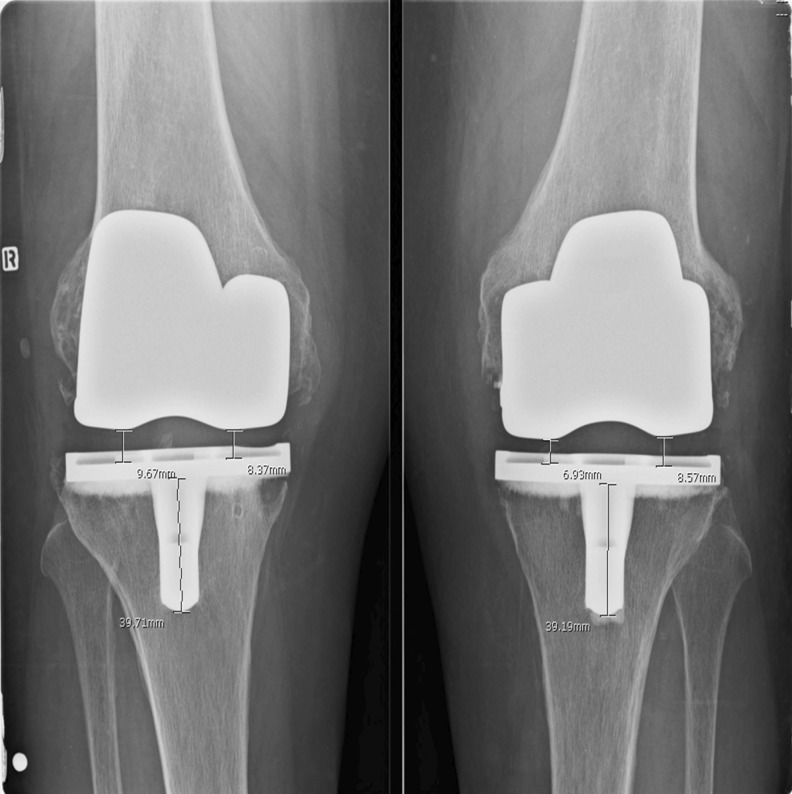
Polyethylene (PE) thickness of medial and lateral compartments measured on anteroposterior radiographs. The estimated amount of linear wear was calculated by deducting the measured thickness, corrected for magnification, from the initial PE thickness, as disclosed by the manufacturer
